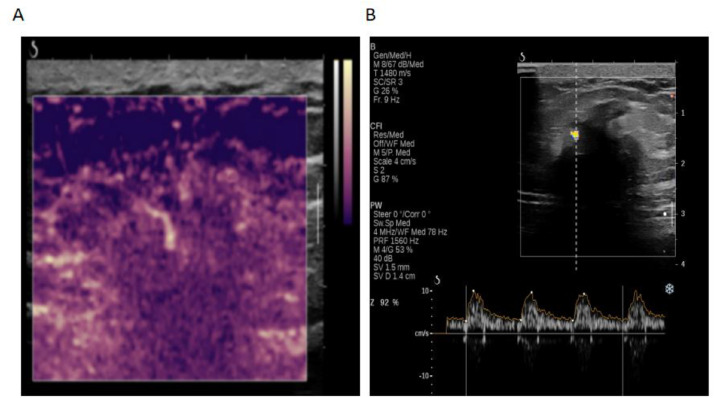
(A) Ultrasound microvascular imaging: Low-velocity microflow within the mass. (B) Pulsed Doppler: Low-velocity arterial-like flow spectrum.
